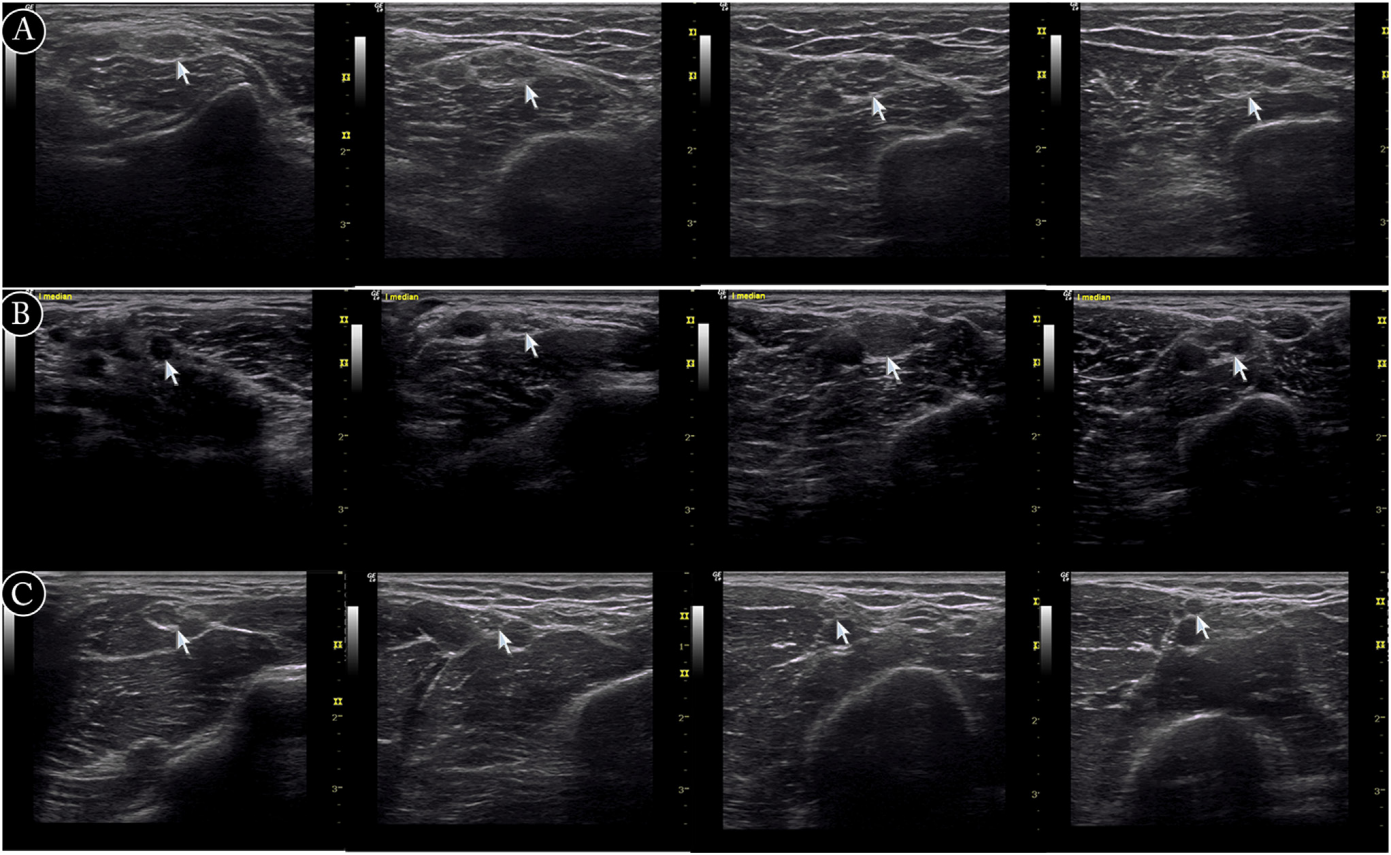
Examples of ultrasound cross-sections showing measurements of cross-sectional area of median nerve in arm. (A) Homogeneous enlargement was shown at sites A7–A10 of median nerve in a Charcot–Marie–Tooth type 1A patient. (B) Segmental enlargement was seen at sites A7–A10 of median nerve in a chronic inflammatory demyelinating polyradiculoneuropathy (CIDP) patient (type 3). (C) Mild nerve enlargement was shown at sites A7–A10 of median nerve in a CIDP patient (type 2).
